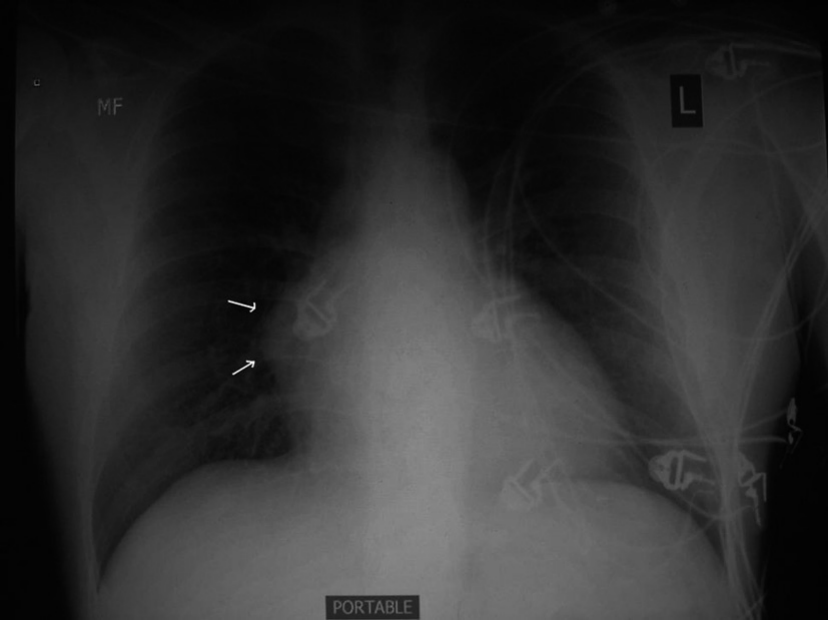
Chest radiograph (postero-anterior view) with calcification in the right heart border (arrows).
